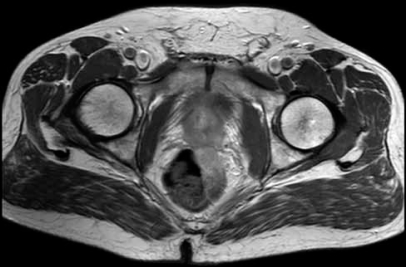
Contrast-enhanced magnetic resonance image demonstrating a tumor mass in the area of the left seminal vesicle, slightly affecting the prostate and the anterior wall of rectum
